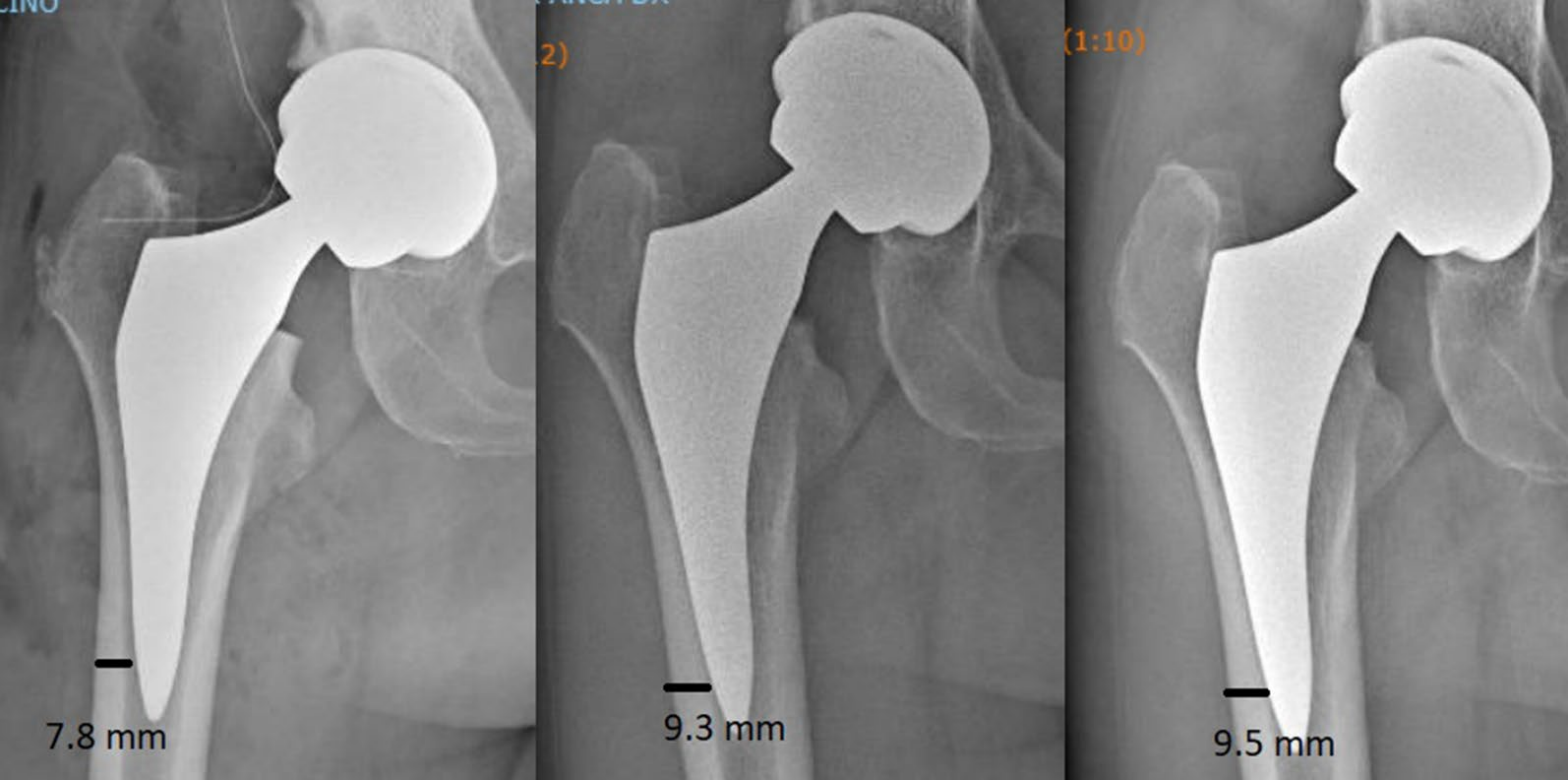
Radiographic change around stem in a 62-year-old patient: postoperative radiograph (left), 1 year follow-up radiograph showing bone cortical hypertrophy in zone 3 (central), 5 years follow-up radiograph with evidence of stabilization of cortical hypertrophy
